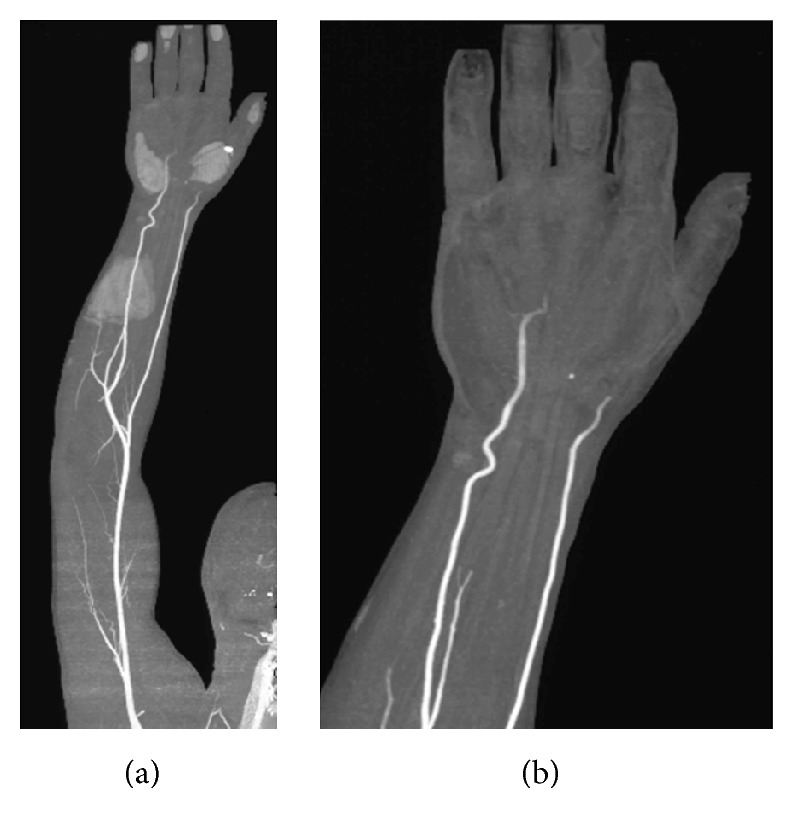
CT angiography of the right upper arm ((a) and (b)). The tridimensional reconstruction (MIP (*Maximum Intensity Projection*) technique) of the arterial circulation showed the interruption of both the radial and ulnar artery flow at level of the thenar and hypothenar eminences and the absence of distal circulation.
